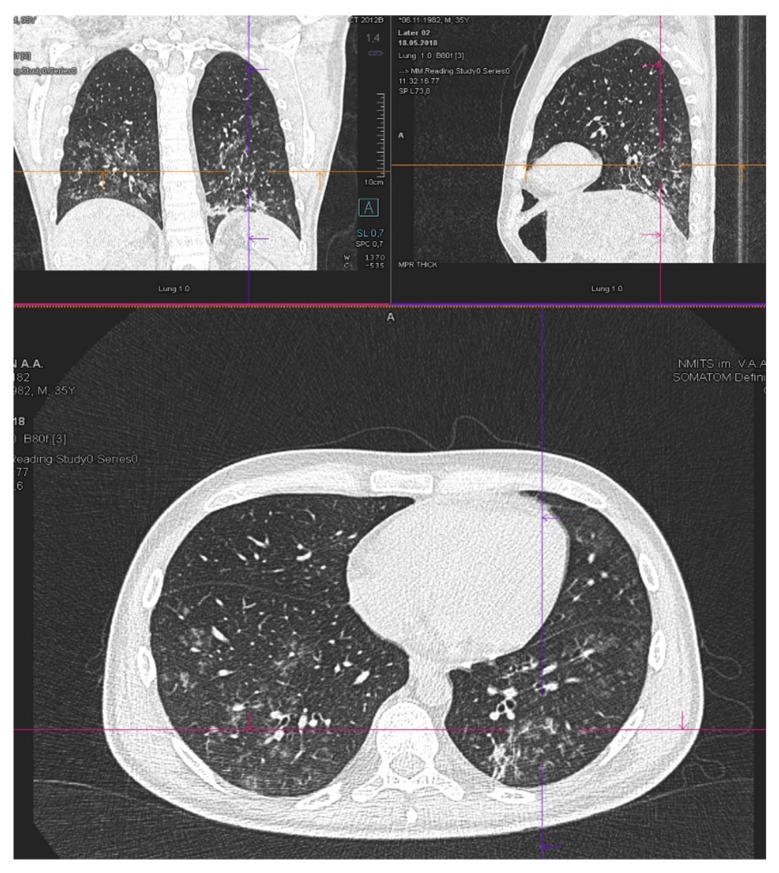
CT chest scan on day 3 of “GO”.
